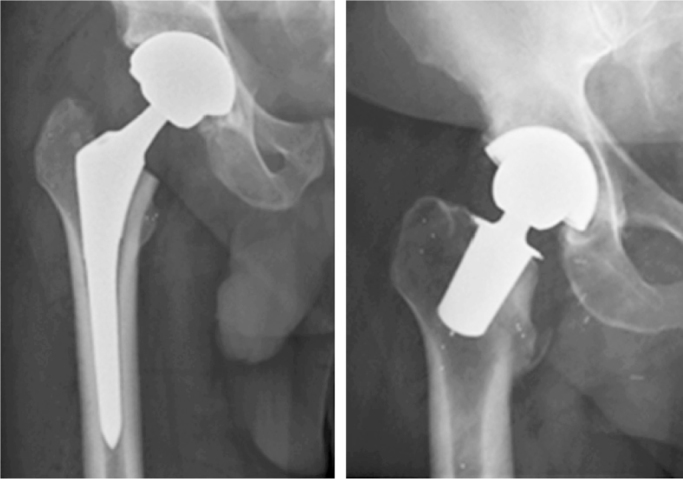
Radiographs of Echo and Primoris total hip arthroplasties.
